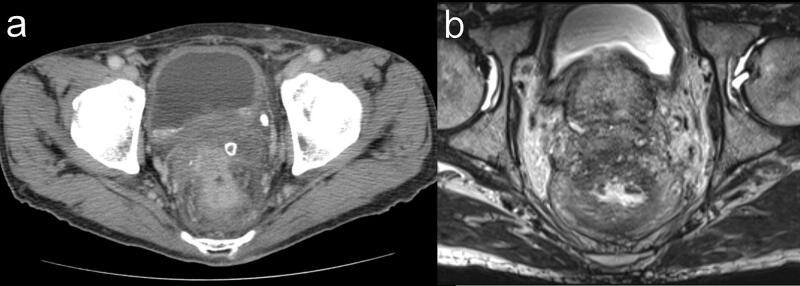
Radiological findings after 4 cycles of panitumumab and mFOLFOX6. A) Abdominal contrast-enhanced computed tomography showing reduced rectal wall thickness. B) Pelvic magnetic resonance imaging showing no indication of the perirectal abscess. mFOLFOX6, modified fluorouracil, leucovorin, and oxaliplatin.
